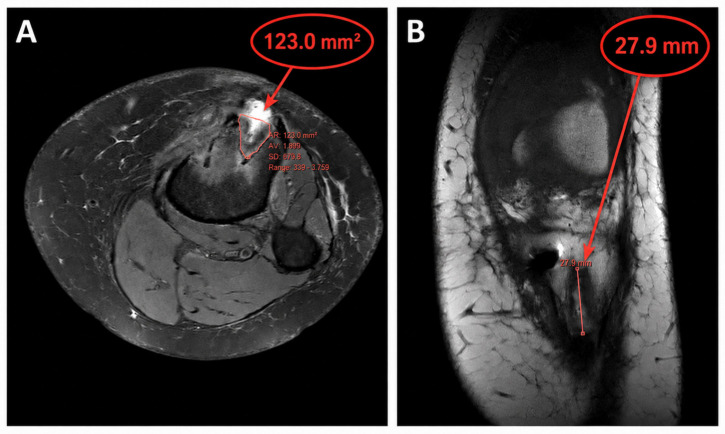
Tibial donor-site bone defect 4 weeks after anterior cruciate ligament reconstruction with a bone–patellar tendon–bone autograft: (A) axial AX PD SPAIR MRI showing the defect area used for base estimation; (B) coronal T1 TSE MRI showing the longitudinal extent of the defect.
